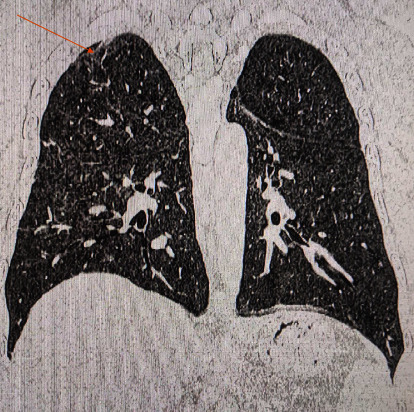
HRCT of the patient chest after completion of therapy (arrow indicates the area of fibrosis).
